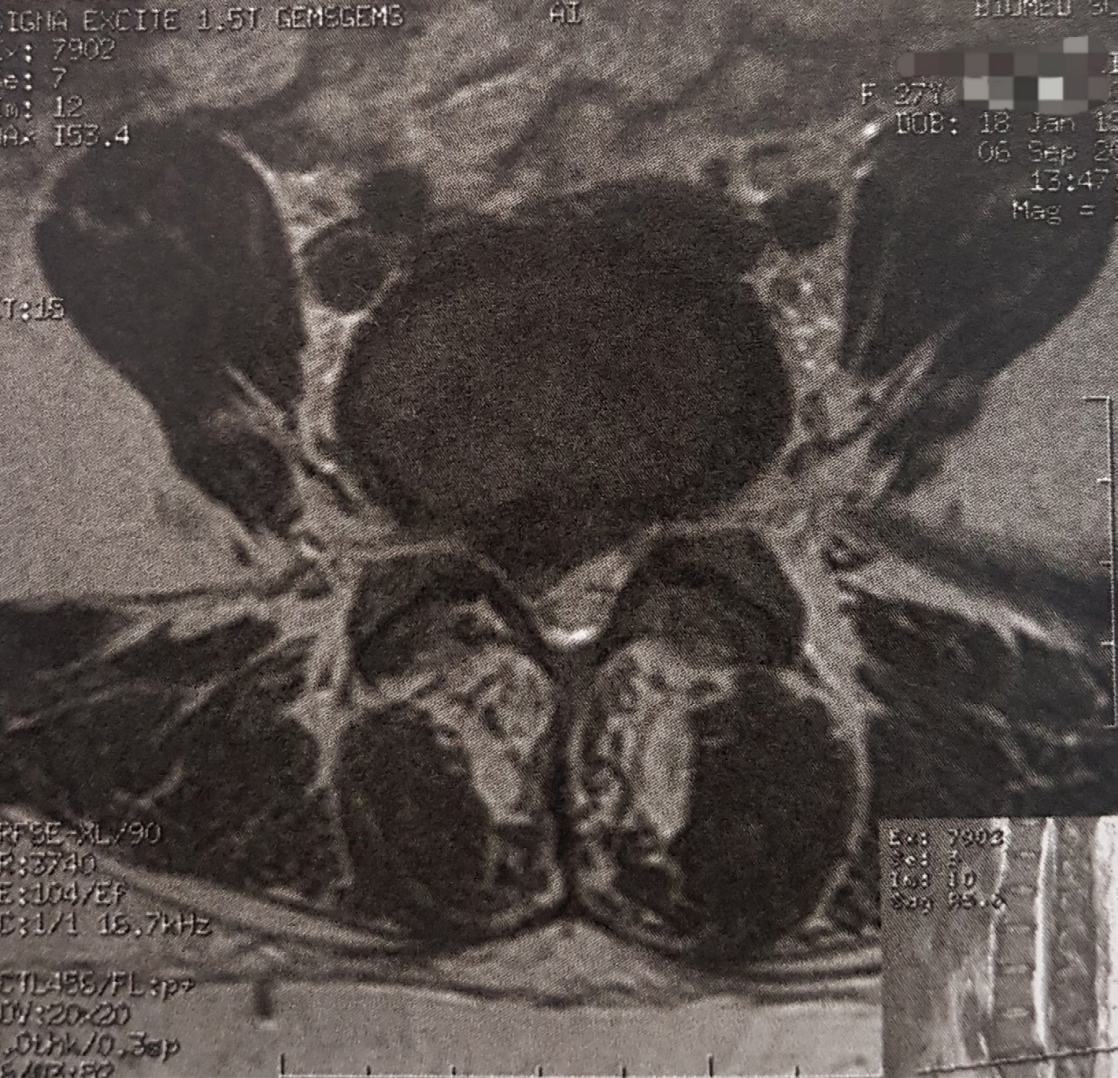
T2-weighted MRI scan, axial section, showing a lumbar disc herniation compressing the right L5 spinal nerve root.
